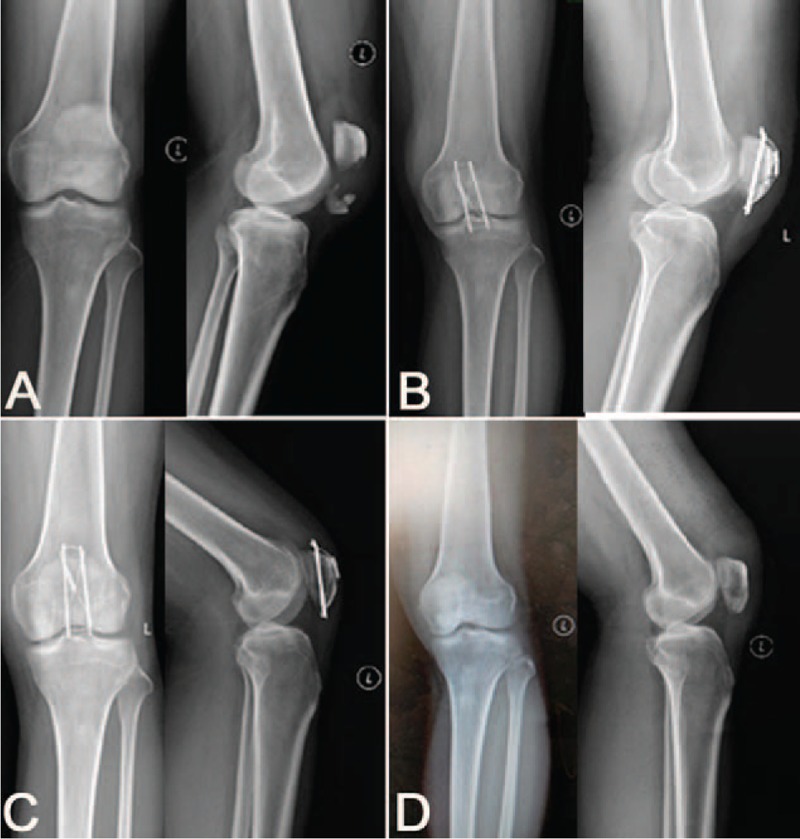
A 36-year-old female patient with left transverse patella fracture underwent MKTB fixation. X-ray film showing transverse patella fracture (A). Postoperative film showing stable fixation of the fragments (B). The fractures got bone union; however, the patient complained of irritating pain caused by relative long tail of the K-wires (C). The pain disappeared after removing implant, showing improvement of the knee function (D). K-wires = Kirschner wires, MKTB = modified Kirschner wire tension band.
